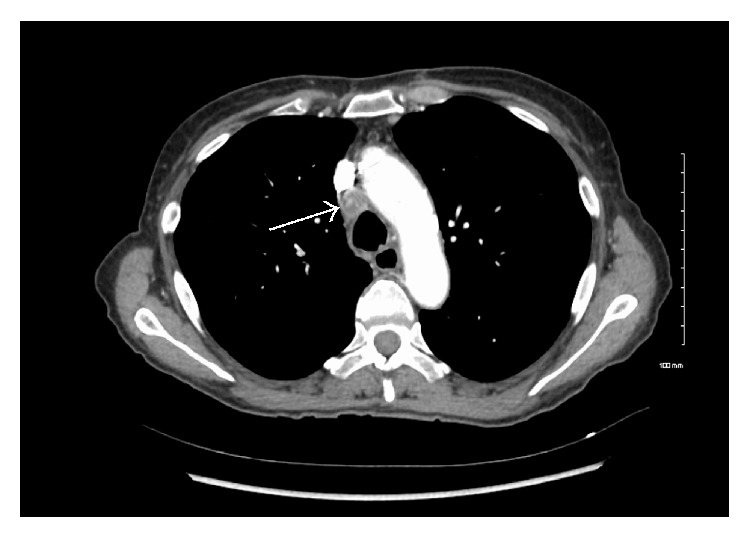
CT scan of the thorax demonstrating necrotic paratracheal node. Enhanced axial CT scan of the thorax, mediastinal window, in a 58-year-old female who presented with bilateral vocal cord immobility of unknown etiology. The positive serum anti-Hu antibody, which is highly associated with small cell lung carcinoma, led to this repeat CT scan. The arrow demonstrates an enlarged level 4R paratracheal lymph node with central necrosis. See Figure 2 for the pathologic description of a biopsy from this node.
